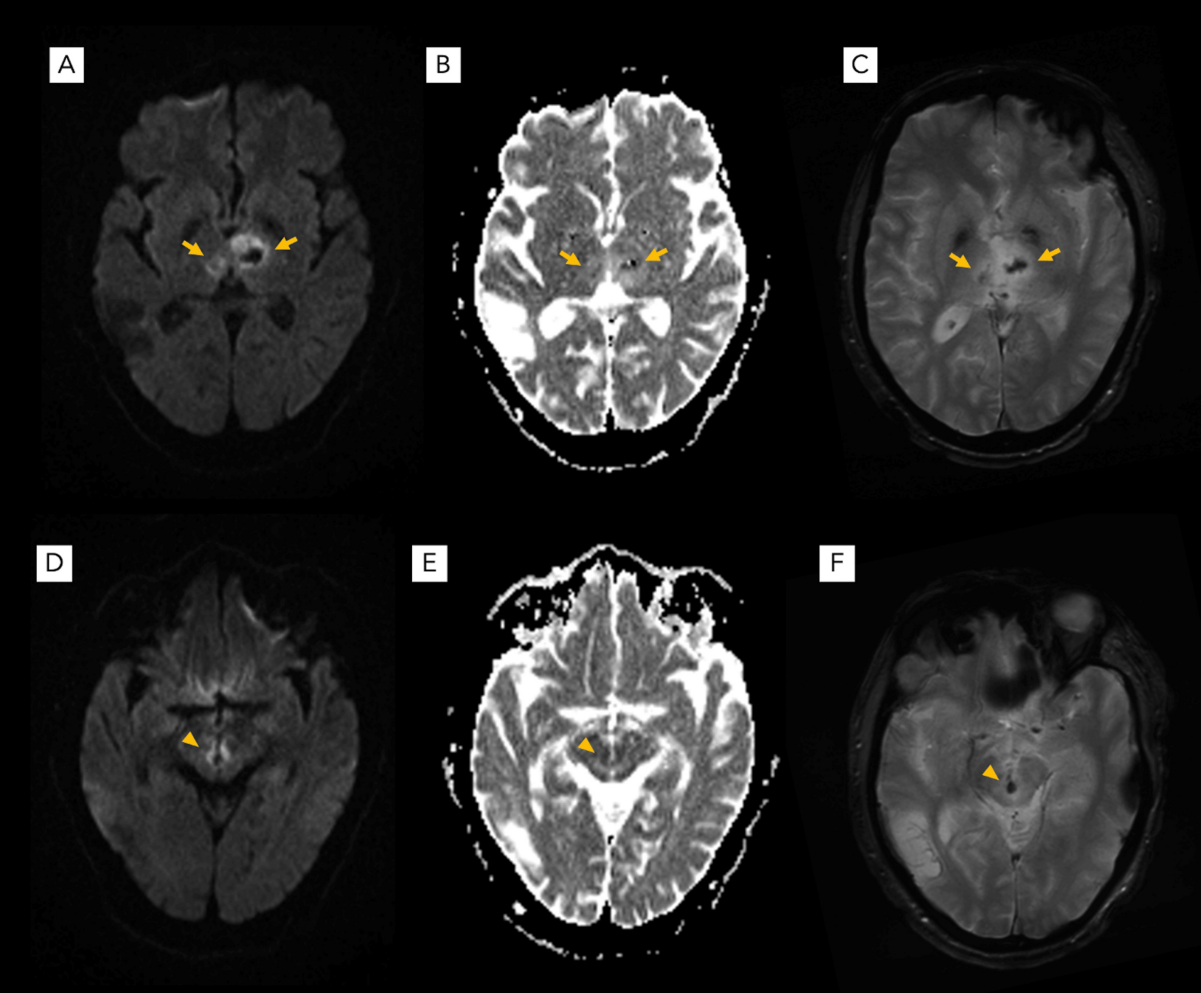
Cranial MRI findings Axial diffusion-weighted (A, D), apparent diffusion coefficient (B, E), and gradient recalled echo (C, F) MRI taken 10 hours post-ictus reveal an acute hemorrhagic infarction of the bilateral thalami (arrows) and rostral mesencephalon (arrowheads).
